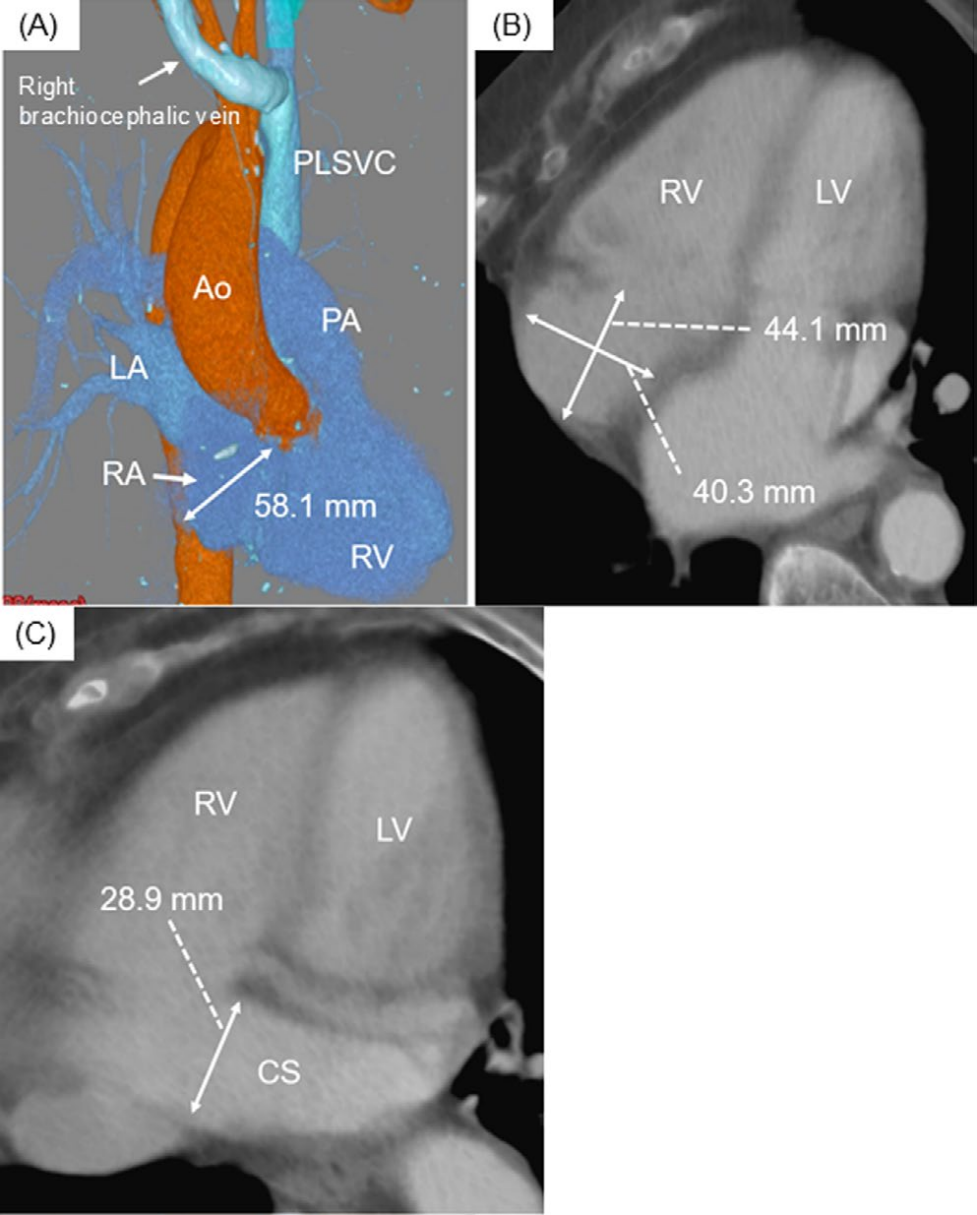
(A) A volume rendering CT image in the RAO view revealed isolated PLSVC and the distance from the IVC outlet to the superior edge of the tricuspid valve annulus is 58.1 mm. (B) The long and transverse diameters of the RA in the horizontal four‐chamber view were 44.1 and 40.3 mm, respectively. (C) The diameter of the dilated coronary sinus was 28.9 mm. Ao, aorta; CS, coronary sinus; CT, computed tomography; IVC, inferior vena cava; LA, left atrium; LV, left ventricle; PA, pulmonary artery; PLSVC, persistent left superior vena cava; RA, right atrium; RAO, right anterior oblique; RV, right ventricle.
